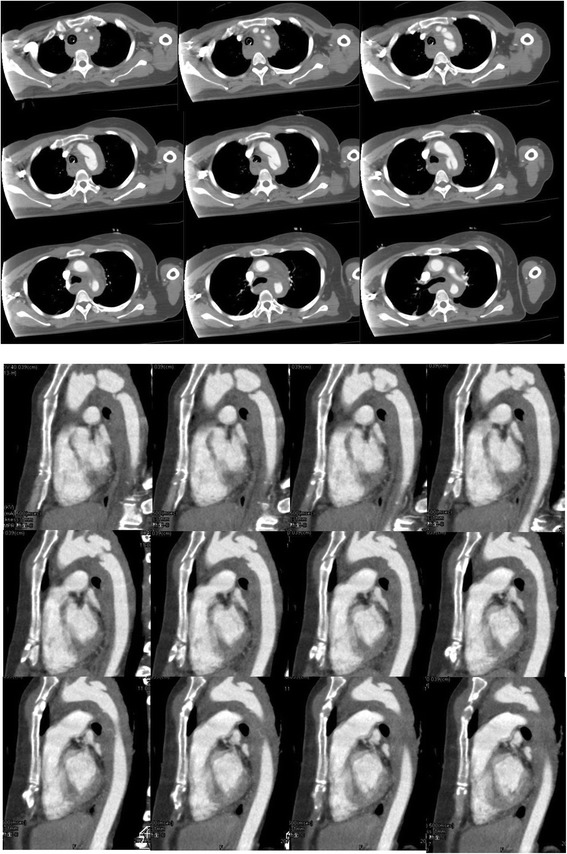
Enhanced CT on admission showed a grade III injury of the thoracic aorta on both axial and sagittal imaging
